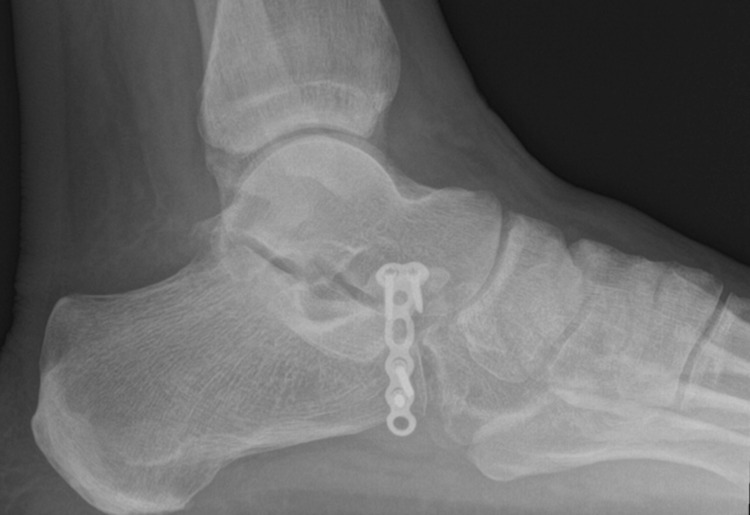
Lateral radiograph of the left foot at four months post-operative
